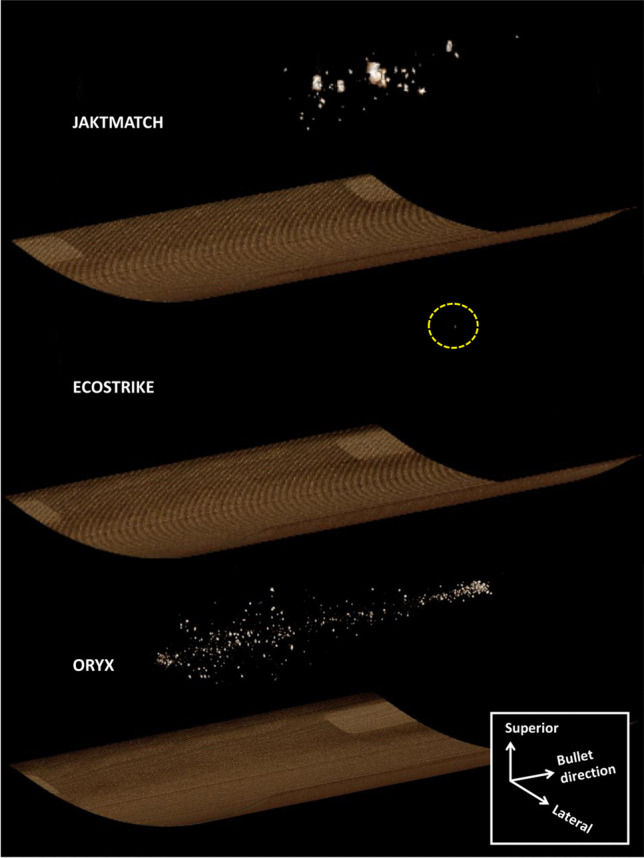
Oblique view of three-dimensional bullet fragment reconstruction based on computed tomography of the gelatine blocks. Dashed circle is used to demarkate the only fragment identified for Ecostrike
